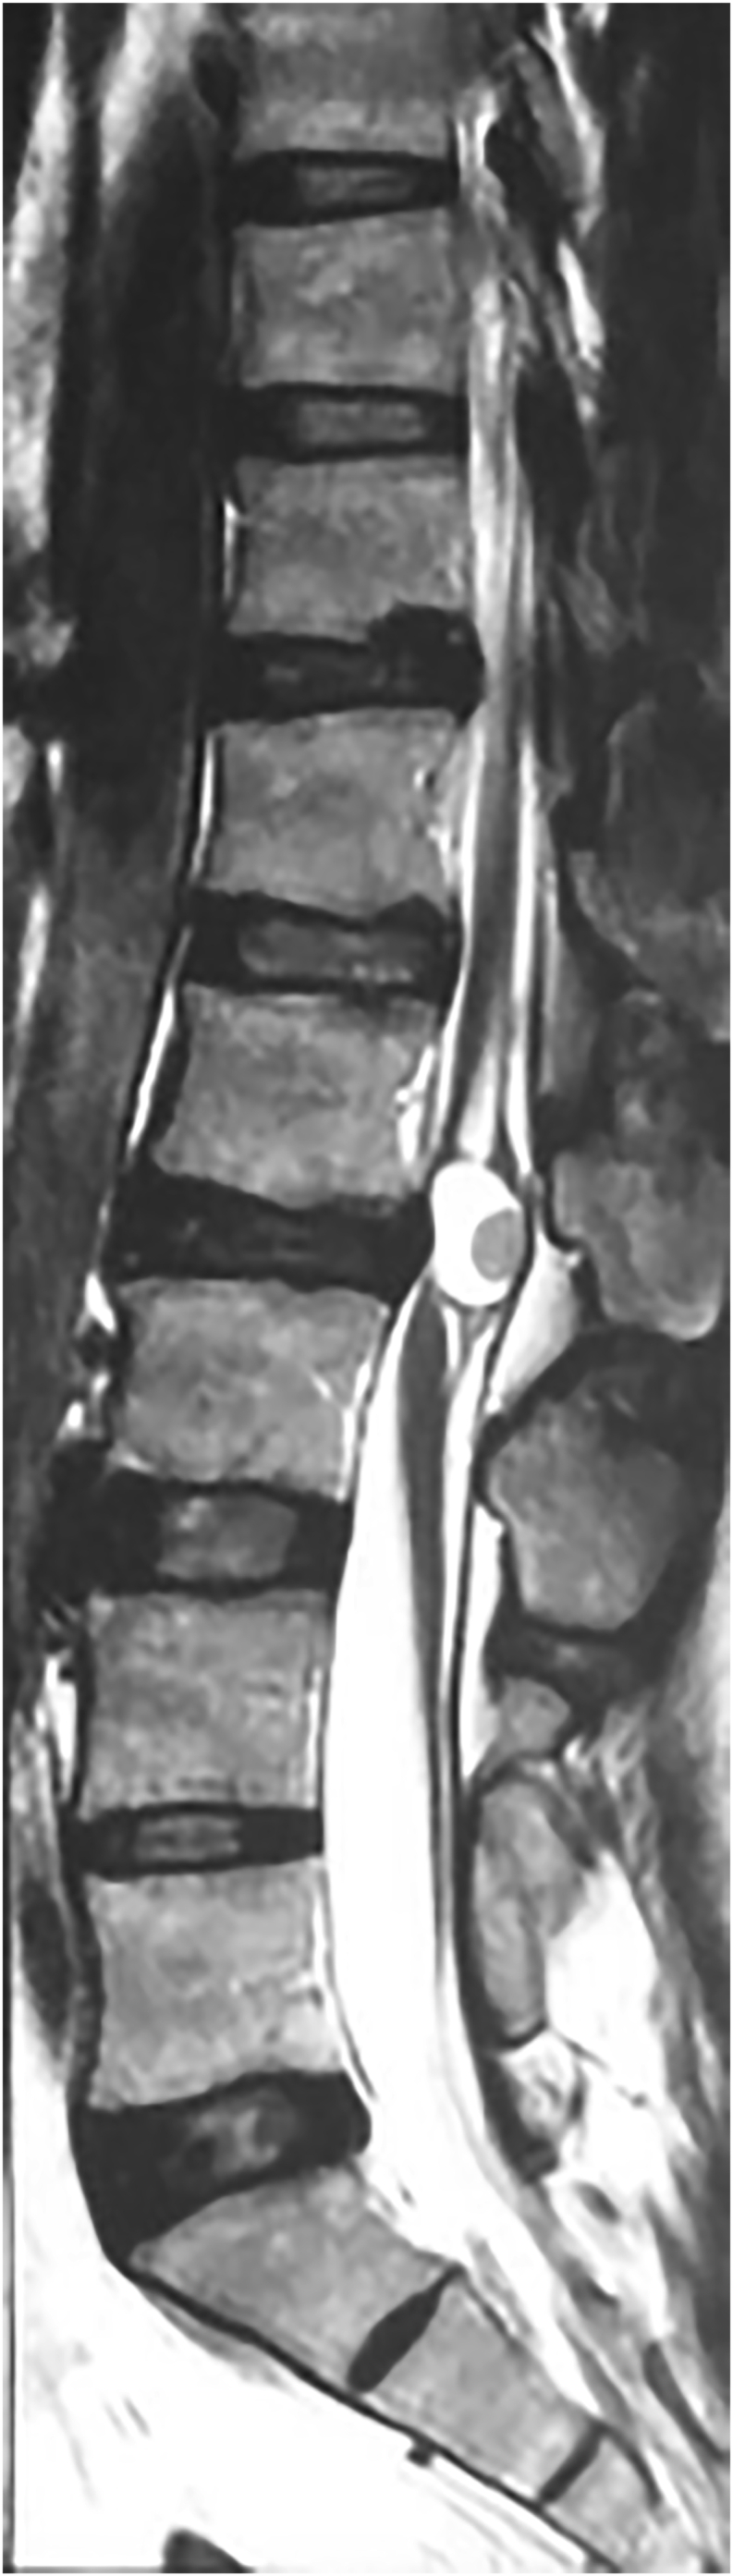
T2-weighted magnetic resonance image showing intradural cystic lesion with an intrinsic nodular component causing compression of the spinal cord.
